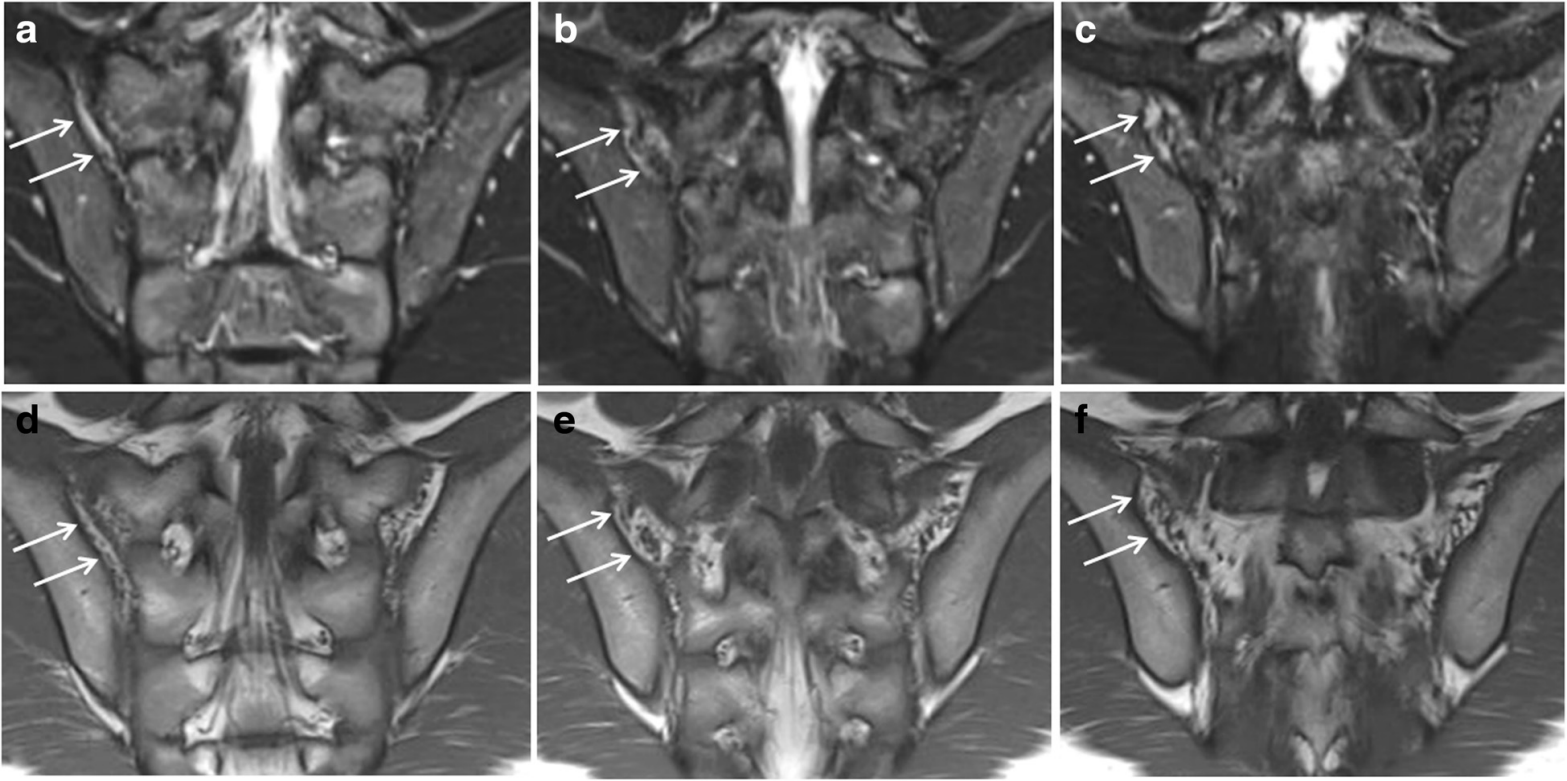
Enthesitis of the retroarticular interosseous ligaments in a 12-year-old boy with enthesitis-related arthritis. a-c. Consecutive semicoronal STIR MR images demonstrate enthesitis of the retroarticular interosseous ligaments on the right side (arrows). d-f. Corresponding semicoronal T1-weighted images demonstrate that the high signal is seen in the retroarticular fat tissue and not in the cartilaginous part of the SI joint
